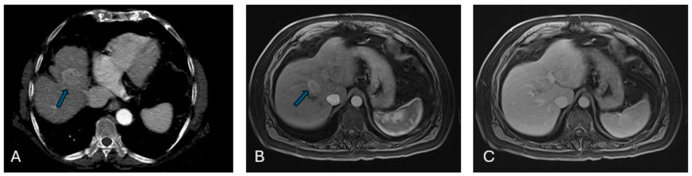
Axial contrast-enhanced computed tomography (CT) and magnetic resonance imaging (MRI) images demonstrating representative hepatocellular carcinoma (HCC) appearances. (A) CT scan from one patient demonstrates heterogeneous enhancement (arrow) in the right hepatic lobe, consistent with HCC. (B) MRI of a different patient demonstrates characteristic arterial hyperenhancement (arrow) of HCC with heterogeneous signal. (C) MRI from the same patient as in panel B demonstrates the characteristic washout of the HCC lesion.
